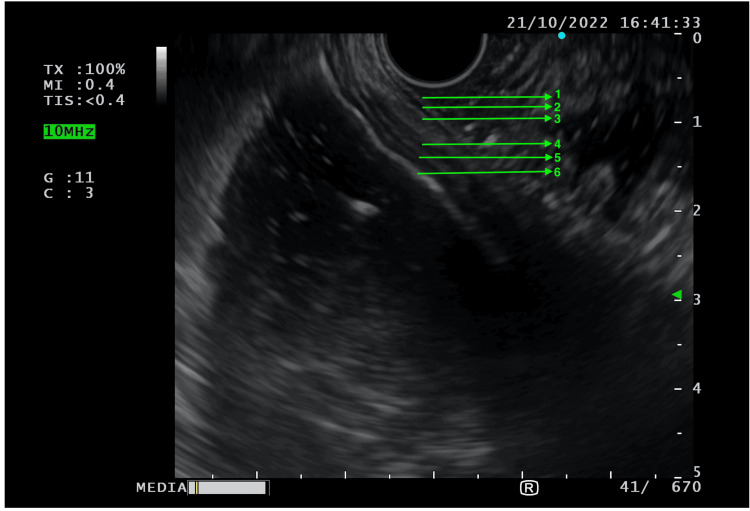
Endoscopic ultrasound (EUS) findings of distal duodenum and jejunum, showing supernumerary subserosal muscle layer 1: Mucosa, 2: Muscularis mucosa, 3: Submucosa, 4: Muscularis externa, 5: Subserosal supernumerary muscle layer, 6: Serosa
